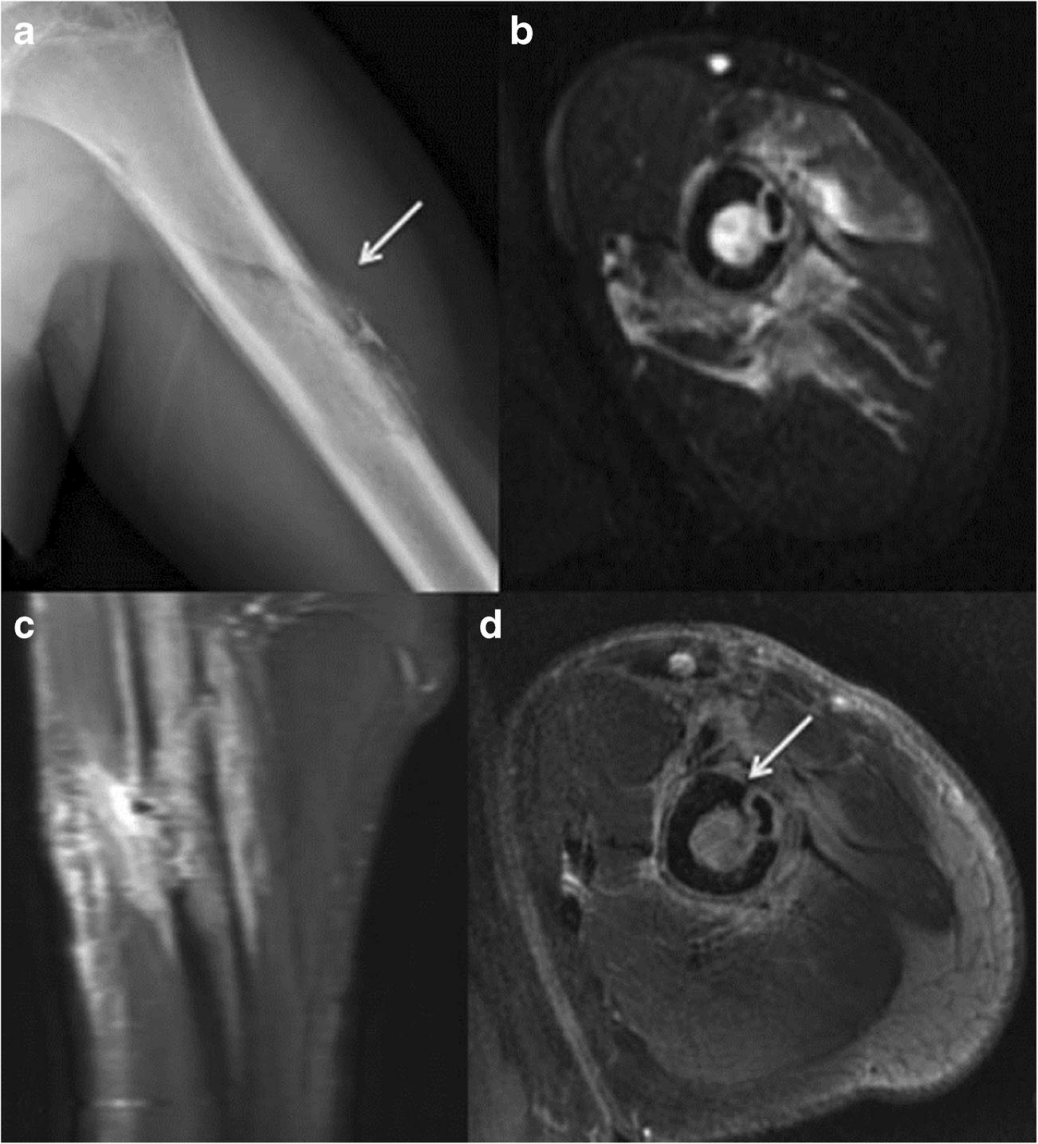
Septic cortical osteitis. Antero-posterior radiograph of the left humerus a shows irregular inhomogenous lamellated periosteal reaction at the left lateral cortical surface of the diaphysis (*thick arrow*). There is an associated obliquely oriented fracture through the proximal humerus. Axial fat suppressed T2 b and coronal fat suppressed T2 c images demonstrate an intracortical hypointensity with surrounding edema through the medullary cavity and the adjacent soft tissues. An axial post-contrast injection image d shows peripheral contrast enhancement surrounding an intracortical hypointensity and enhancement through the medullary cavity and surrounding soft tissues (*thin arrow*)
